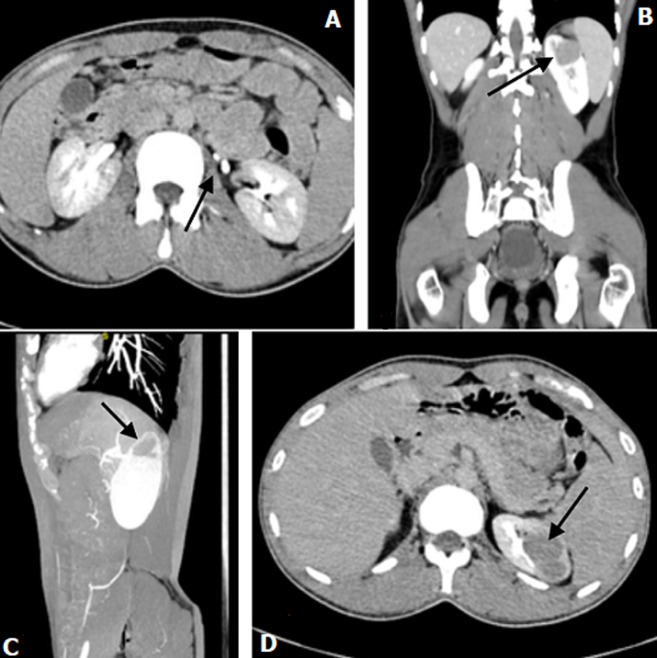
CT imaging of duplex kidney and renal tumor (arrow); axial view showing two ureters (arrow) (A); renal mass in the upper pole of the left kidney in coronal view (arrow) (B); in sagittal view (arrow) (C); in axial view (arrow) (D)
